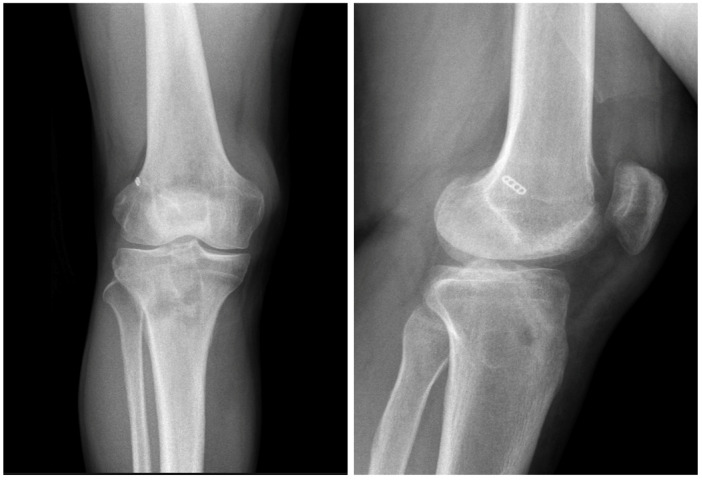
Postoperative AP knee radiographs after conventional ACL reconstruction.
